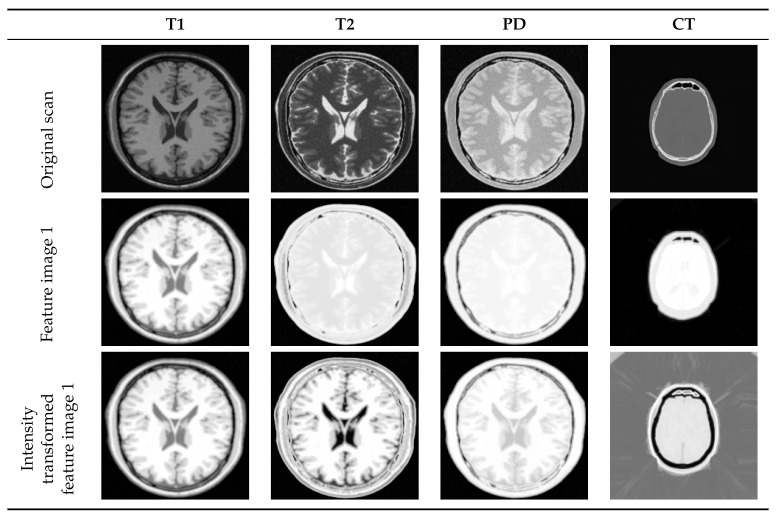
Intensity transformation of multi-modal medical scans. (Top row) shows the original MRI and CT scans of the human brain; (Middle row) shows the first feature image of each modality after manifold learning; (Bottom row) shows the same feature images after manifold alignment. Intensity mapping of feature images obtained from T2-, PD-MRI, and CT are matched with the one obtained from T1-MRI.
